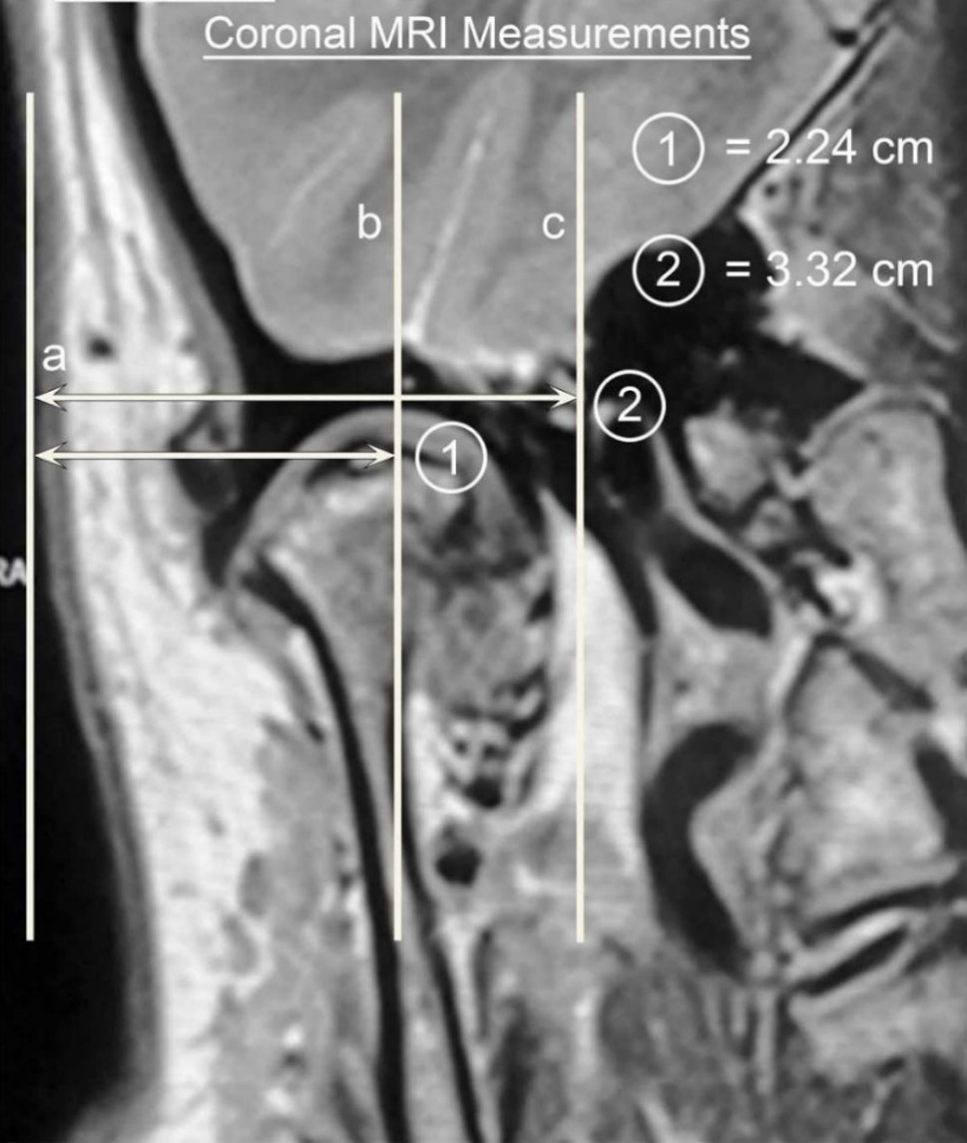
Coronal measures superimposed on the MRI
